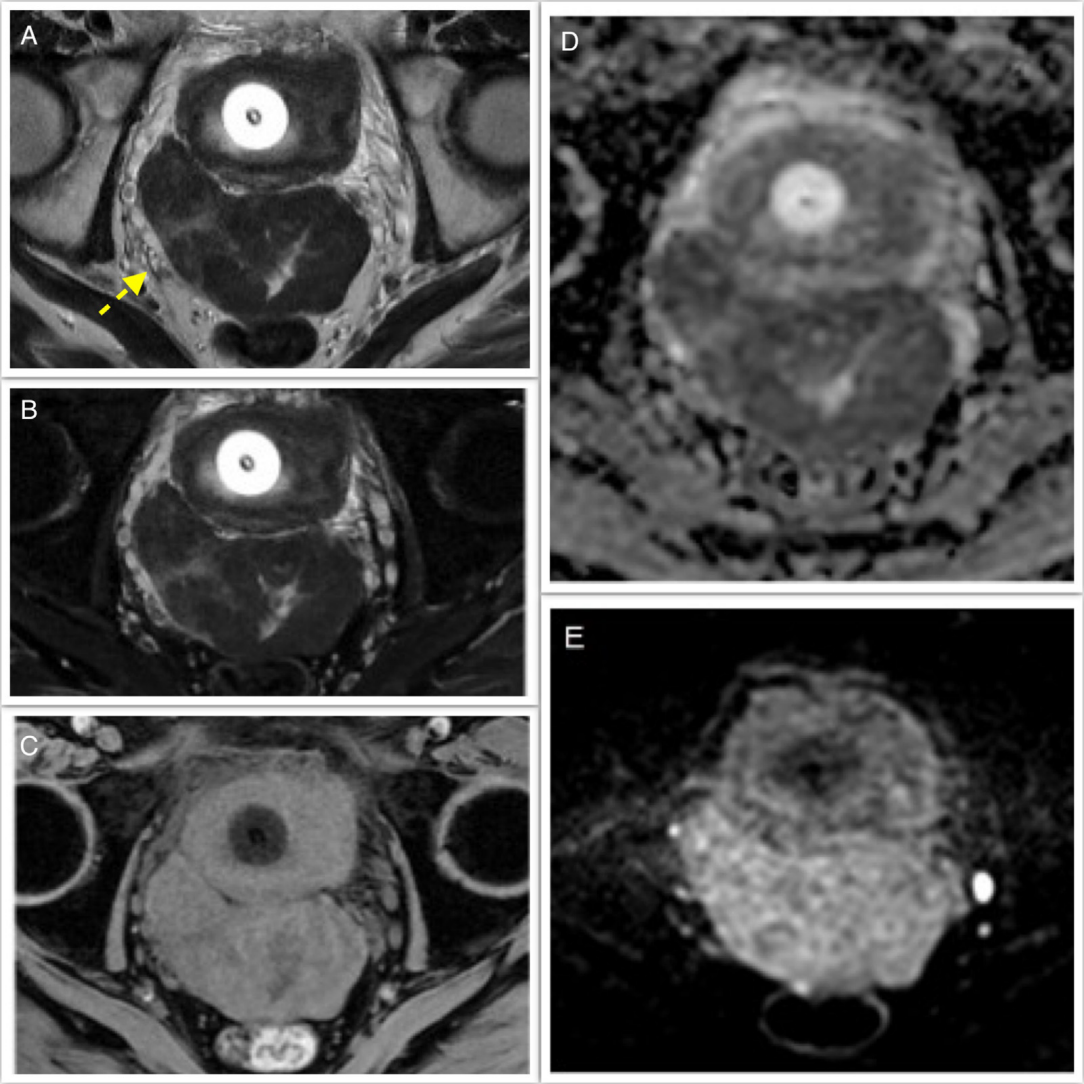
Axial Pelvic MRI T2-w with (A, arrow) and without (B) fat suppression, T1-w fat saturation (C) and DWI with ADC map (D-E), show a slight inhomogeneity of the lesion, that exhibit an hypointensity on T2, an hypointensity on T1 and a restriction of diffusion.
